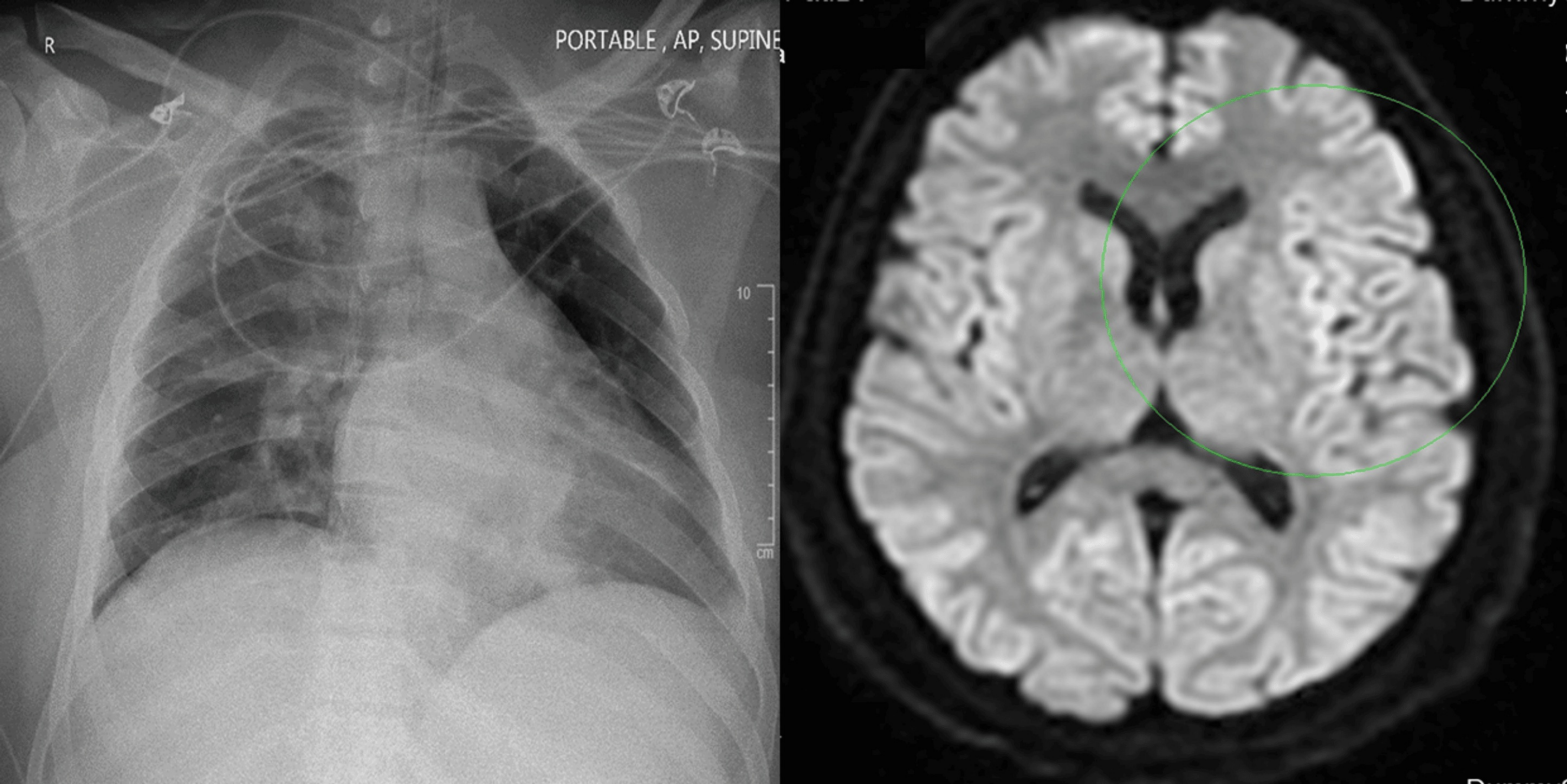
Imaging studies of the patient The picture on the left shows Chest X-ray demonstrating blunted left costophrenic angle. The picture on the right is MRI showing left fronto-parietal cortical subtle hyperintense diffusion-weighted imaging (DWI)–fluid-attenuated inversion recovery (FLAIR) signal (green circle)
